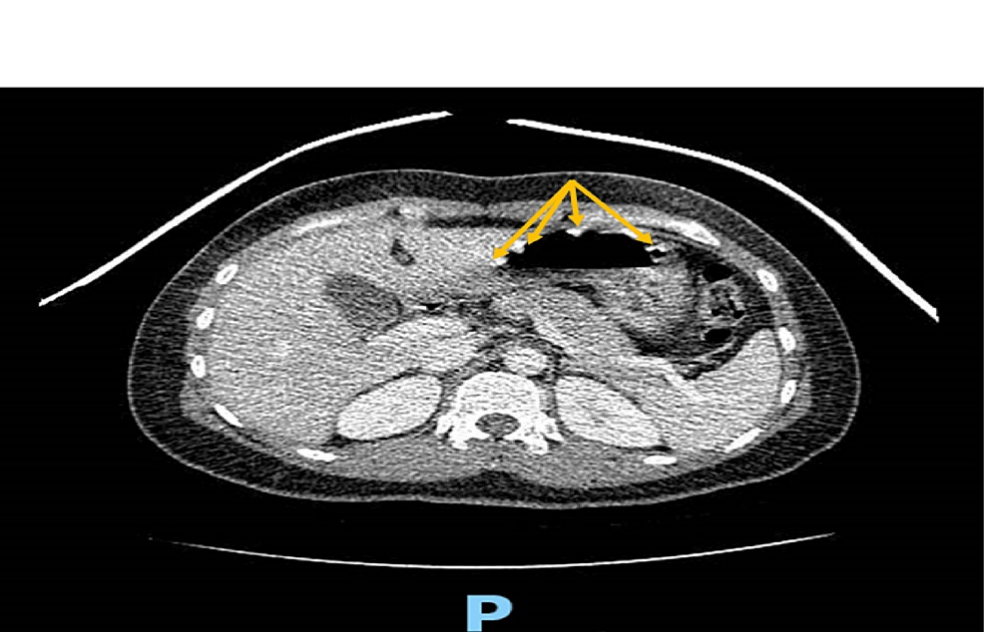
CT scan of the abdomen showing four hyperdense substances (yellow arrows) in the stomach.
